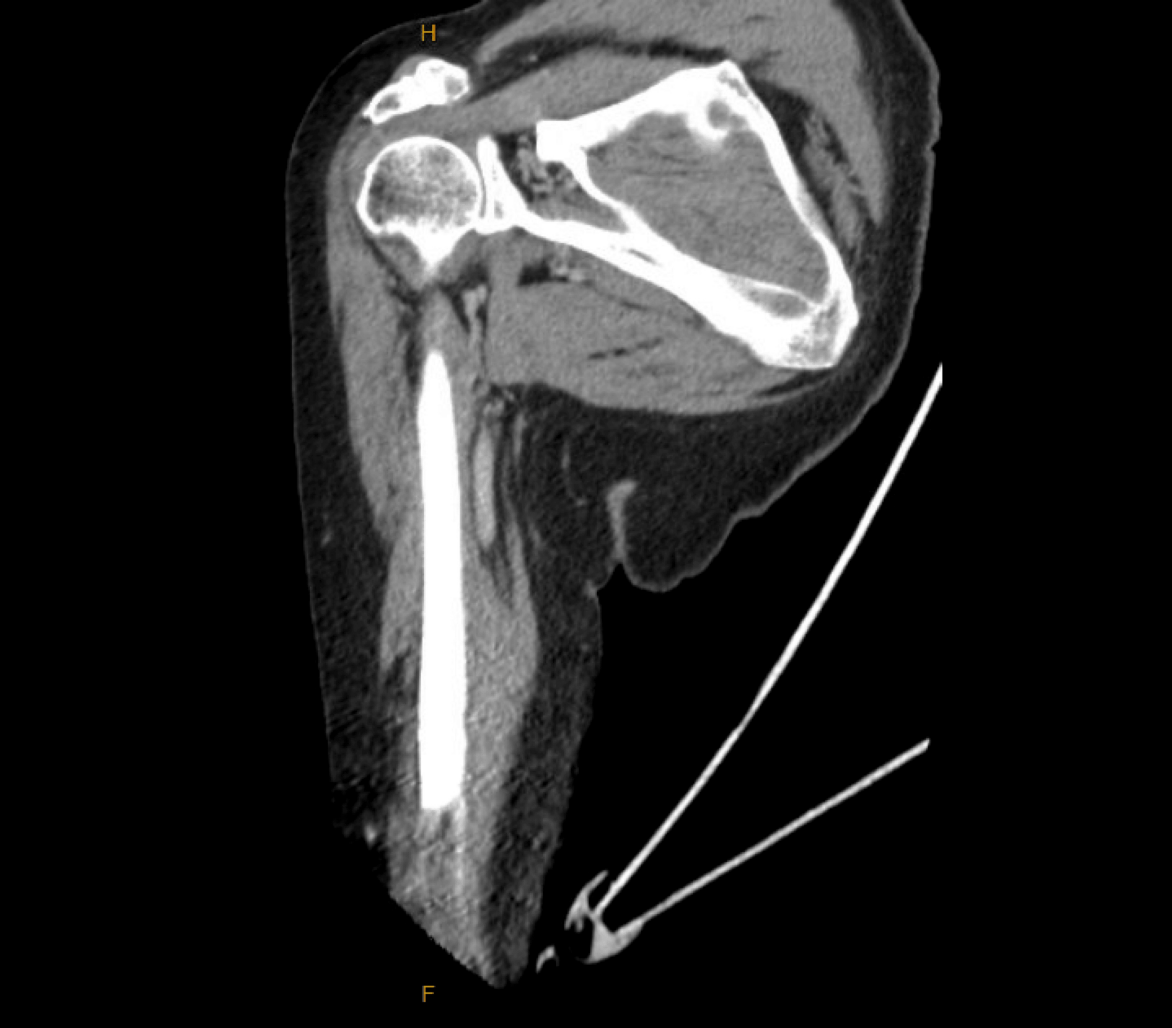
Coronal CT Soft Tissue Window Image of the Right Humerus With IV Contrast Coronal CT image of the right humerus in soft tissue window (with IV contrast), demonstrating preserved soft tissue planes and musculature without evidence of acute pathology. The deltoid and rotator cuff muscles appear intact with normal bulk and symmetry. No soft tissue mass, organized fluid collection, or joint effusion is visualized. The axillary region shows no lymphadenopathy, and the neurovascular bundle appears unremarkable. The acromioclavicular and glenohumeral joints are partially visualized and demonstrate chronic degenerative changes without acute abnormalities.
